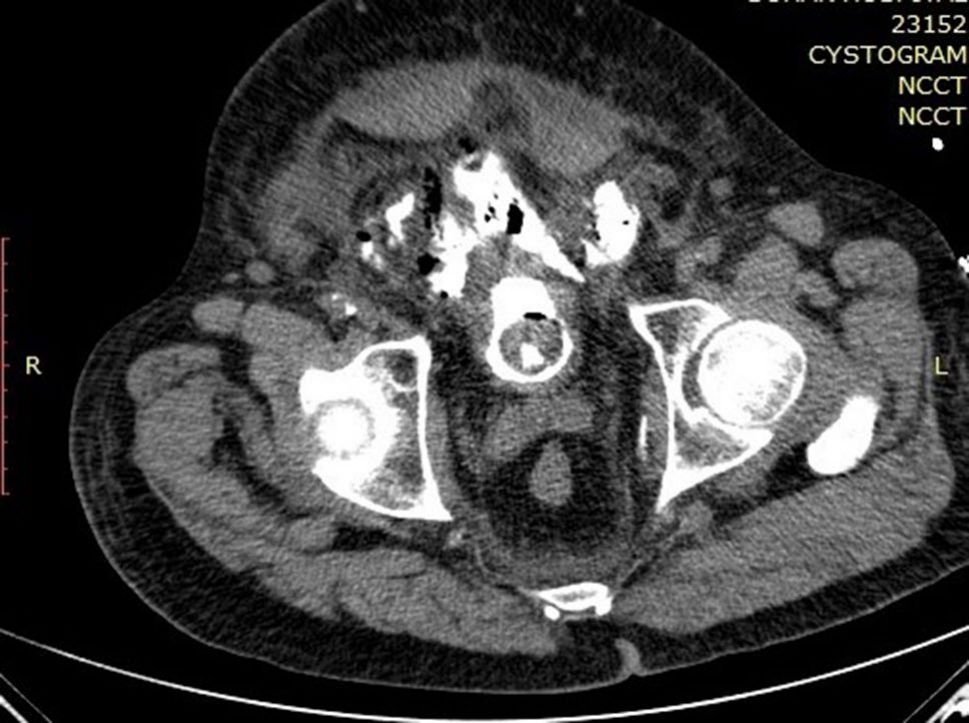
65-year-old male who came to the ER with an acute abdomen 5 days post TURBT and after 2 days of removal of the urethral catheter. CT cystogram axial view showing extravasation of the contrast outside the UB in the extraperitoneal space
